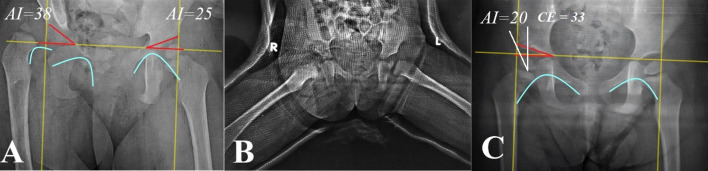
Female child, 18 months, presented with right DDH, Tönnis grade III. A preoperative. B Immediate postoperative. C After 20 months of follow-up, the radiographic result was Severin’s grade I
